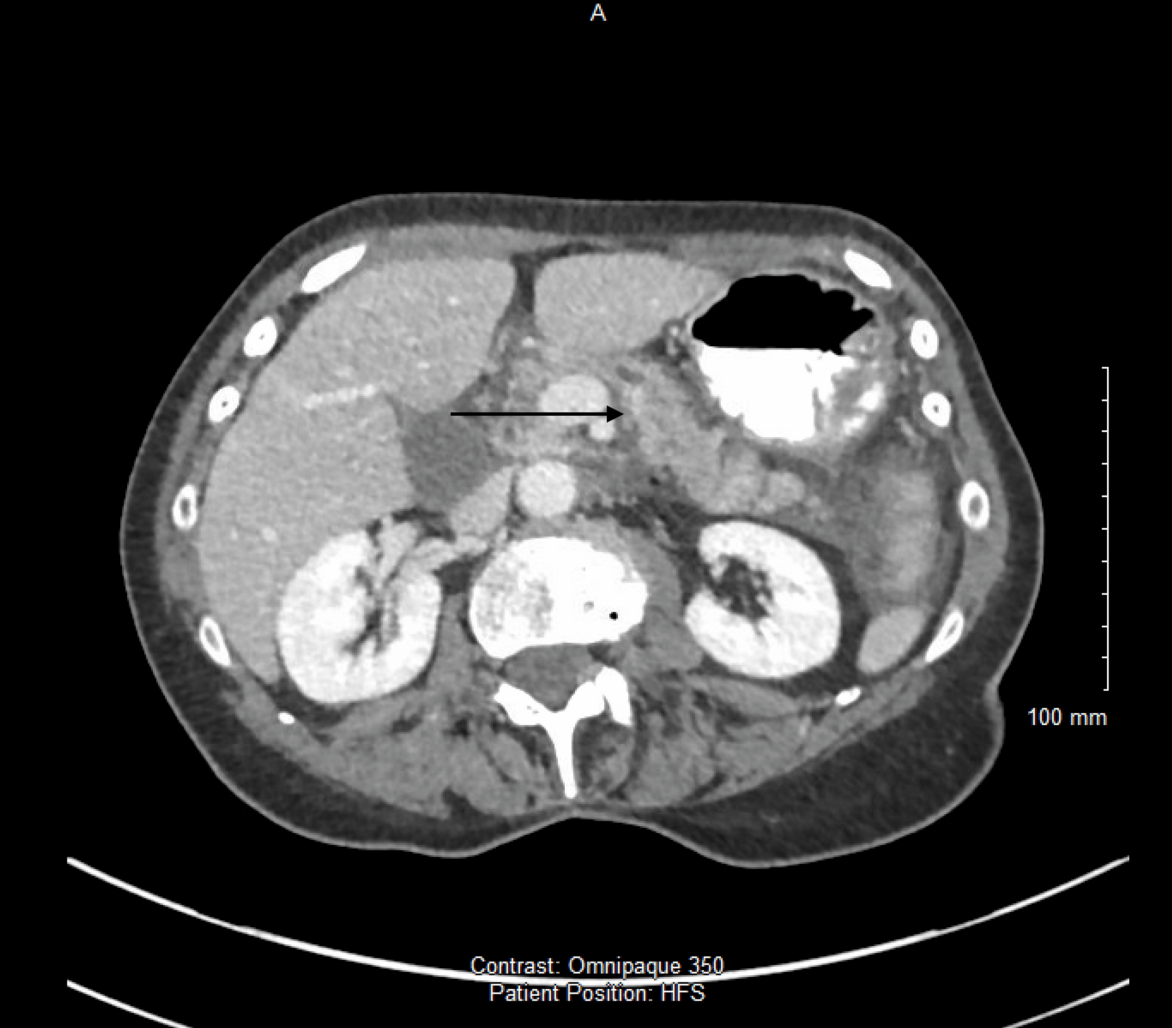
Axial contrast-enhanced CT image of the upper abdomen demonstrating peripancreatic fat stranding and inflammatory changes centred on the pancreatic head and second part of the duodenum (arrow)
